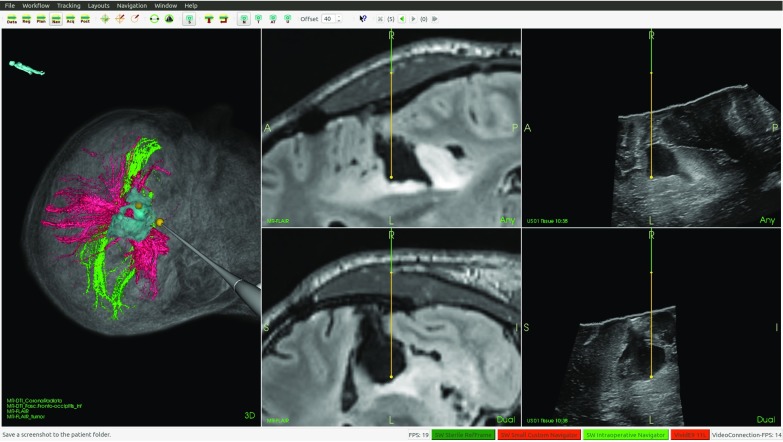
Intraoperative navigation using MR FLAIR and 3D US during resection of a low-grade glioma
